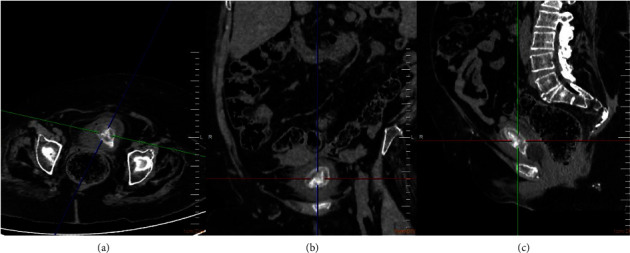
Pelvic CT images (axial (a), coronal (b), and sagittal sections (c)) showing an amorphous dense oval mass in the bladder.
